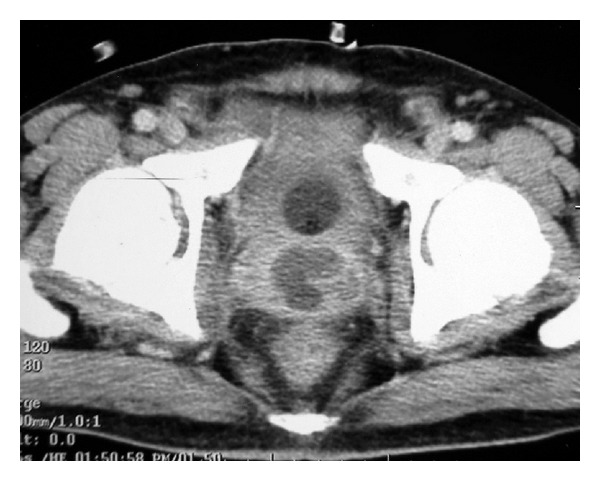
Contrast-enhanced CT image of a patient showing multiloculated prostatic abscesses involving almost whole of the prostate. Patient with prior failed TRUS-guided aspirationgo responded well after TURP.
